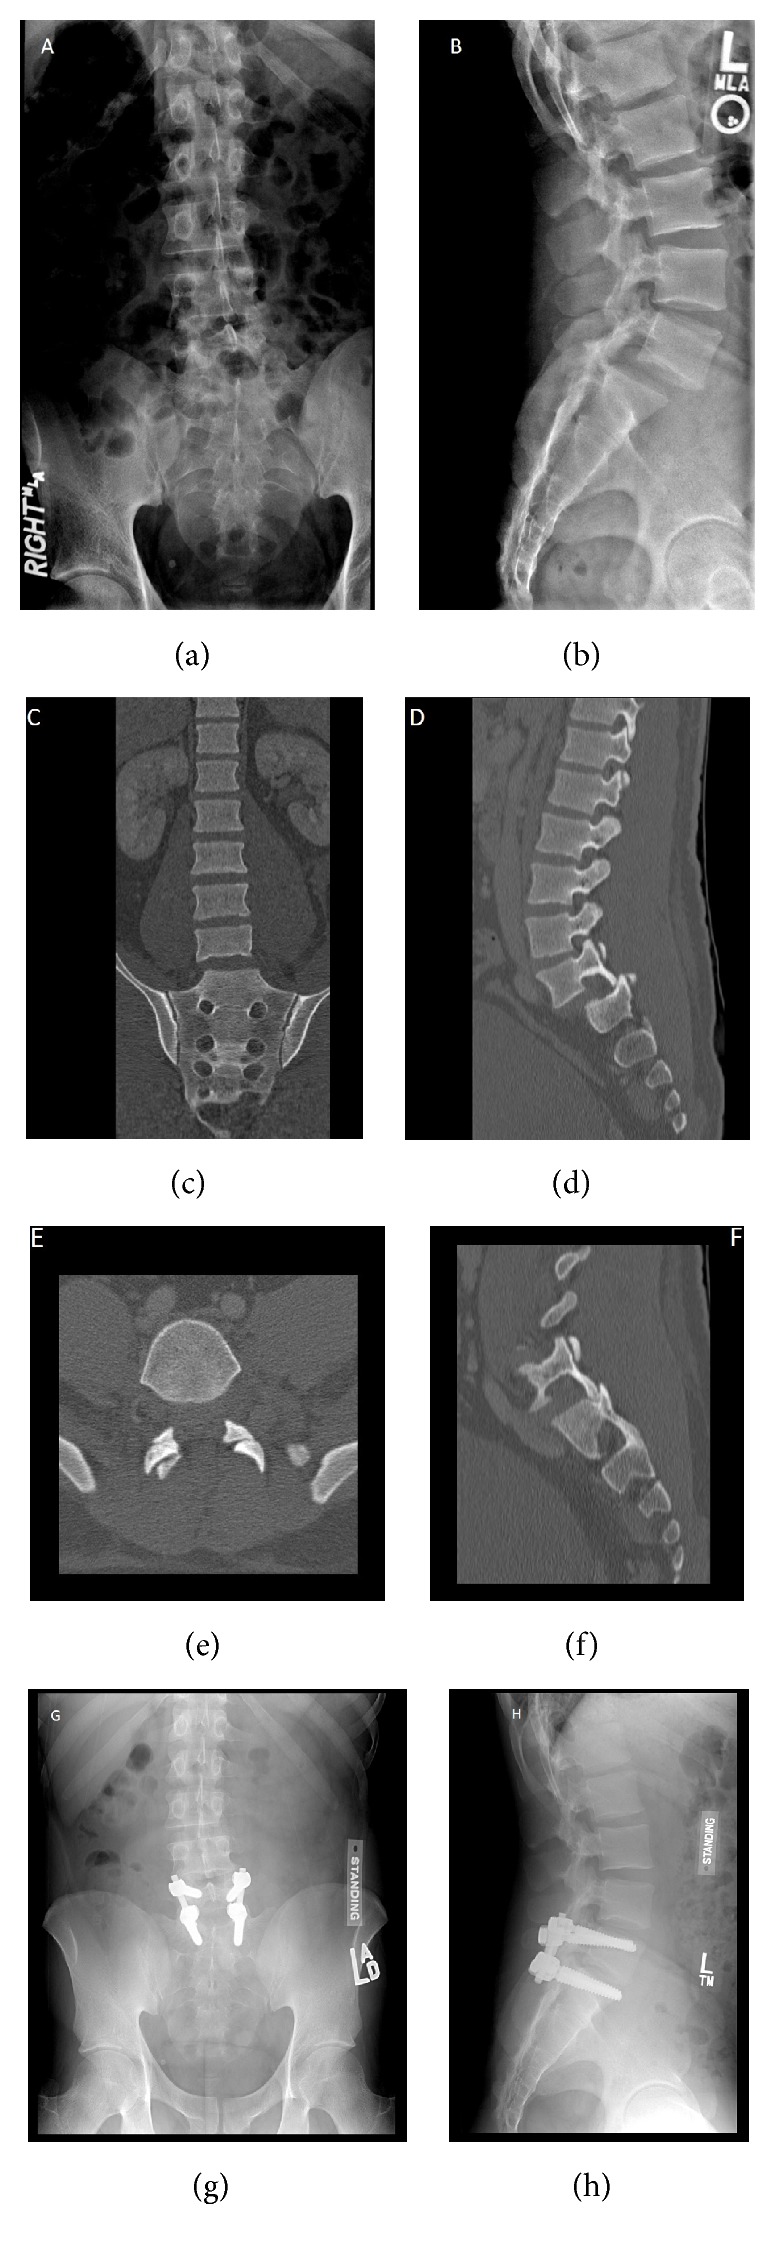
Imaging of a 25-year-old male patient who was involved in an all-terrain vehicle accident. He was ejected from the vehicle and presented with low back pain and intermittent bilateral lower extremity radicular pain with paresthesia. Figures (a) and (b) demonstrate anteroposterior and lateral radiographs, respectively. Coronal CT shows minimal lateralization of L5 over S1 (c). Sagittal view shows anterior dislocation of L5 over S1 with jumped facets (d). Axial (e) image cut through the same level as the sagittal image (f) shows bilateral jumped facets at L5-S1. The patient underwent posterior spinal instrumentation and fusion of the L5 and S1 vertebrae using pedicle screws and rods. Postoperative anteroposterior (g) and lateral (h) images demonstrate a reduced L5-S1 joint. (Courtesy of University of Alabama at Birmingham, Department of Orthopaedic Surgery, Spine Fellowship Program, Birmingham, Alabama, USA).
